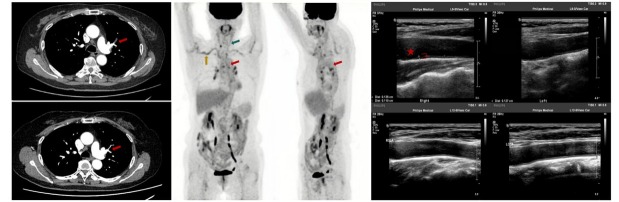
Integrated imaging findings of pulmonary involvement and large-vessel inflammation in GCA. (A) Pulmonary imaging on CTPA before and after treatment. Before treatment (upper panel): CTPA identified a filling defect in the left upper lobe pulmonary artery, consistent with pulmonary embolism; After treatment (lower panel): CTPA demonstrated complete resolution of the pulmonary embolism, with no residual filling defects and only mild stenosis remaining in the upper lobe branches of the left pulmonary artery. (B) PET-CT findings in GCA. The coronal plane (left) and sagittal plane (right) PET-CT images show increased radioactive uptake in the aorta (red arrows), bilateral subclavian and axillary arteries (yellow arrows), and bilateral common carotid arteries (green arrows), consistent with large-vessel inflammation. (C) Vascular ultrasound of the carotid arteries before and after treatment. Before treatment (upper panel): Carotid vascular ultrasound shows diffuse, concentric wall thickening of the common carotid artery, consistent with active large-vessel vasculitis; After treatment (lower panel): Follow-up vascular ultrasound shows marked improvement, with no evident arterial wall thickening after prednisone and tocilizumab therapy.
